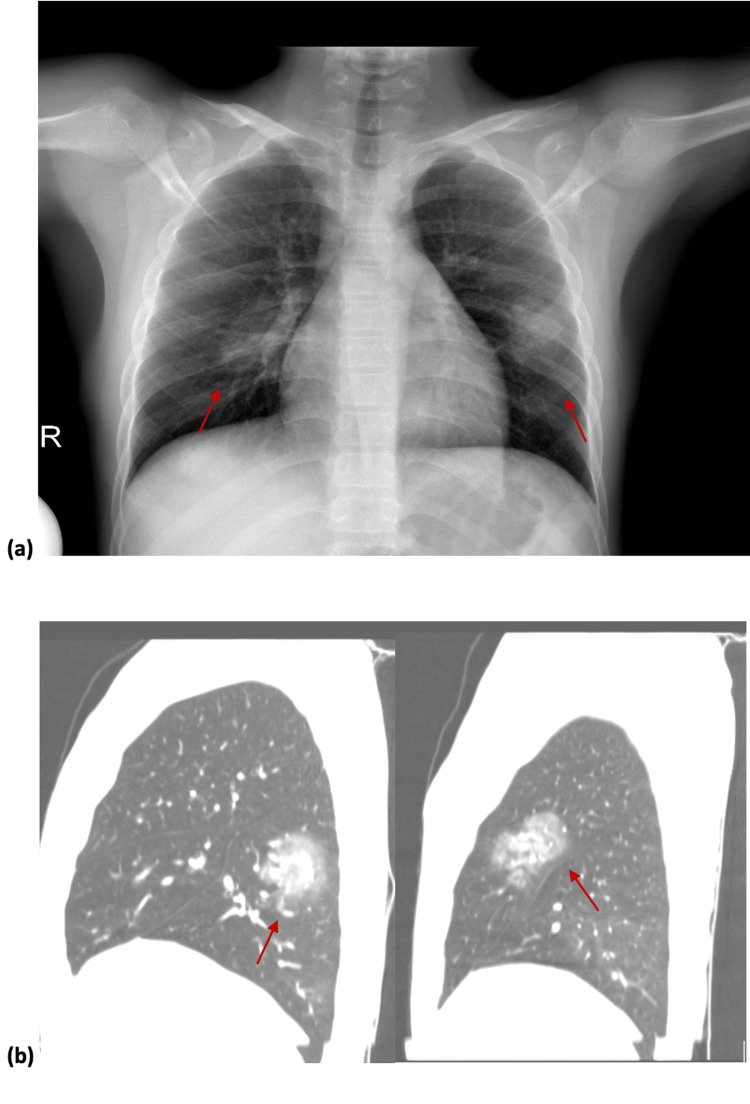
(a) Chest X-ray on admission showing patchy shadows in both lungs, (b) lung CT (sagittal plane) showing ground-glass patches on both lungs.
